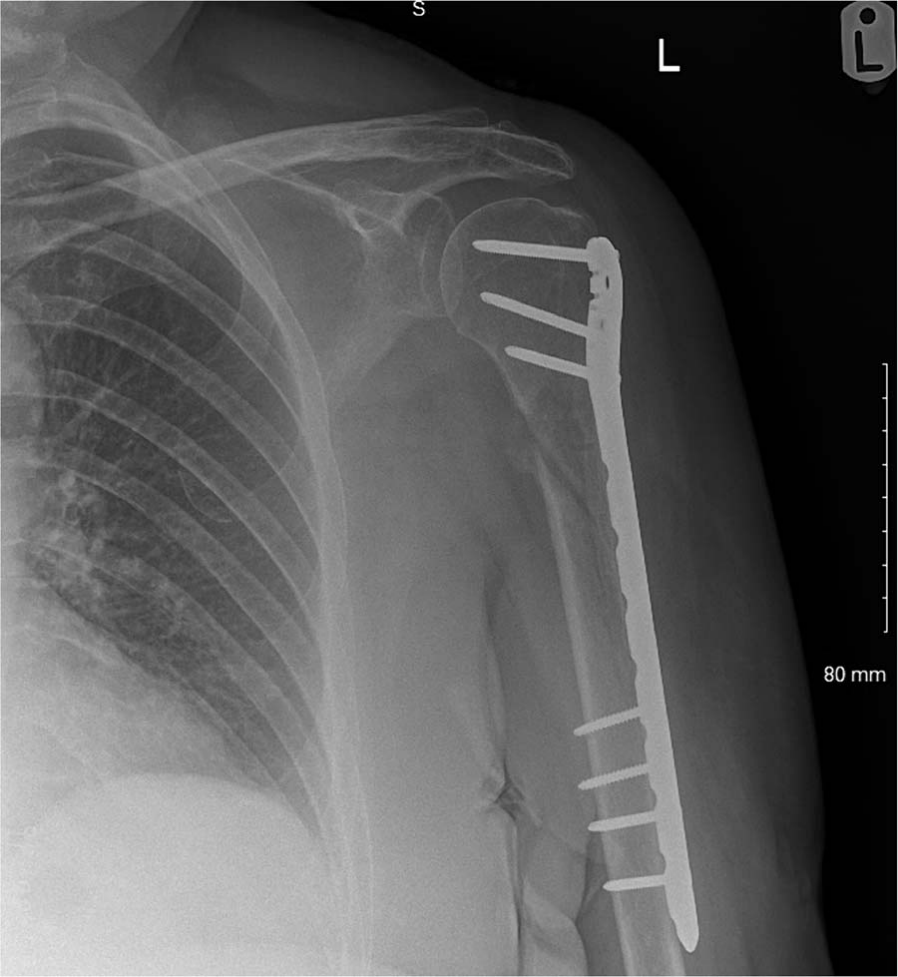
Post-operative radiographs following humerus fixation.
